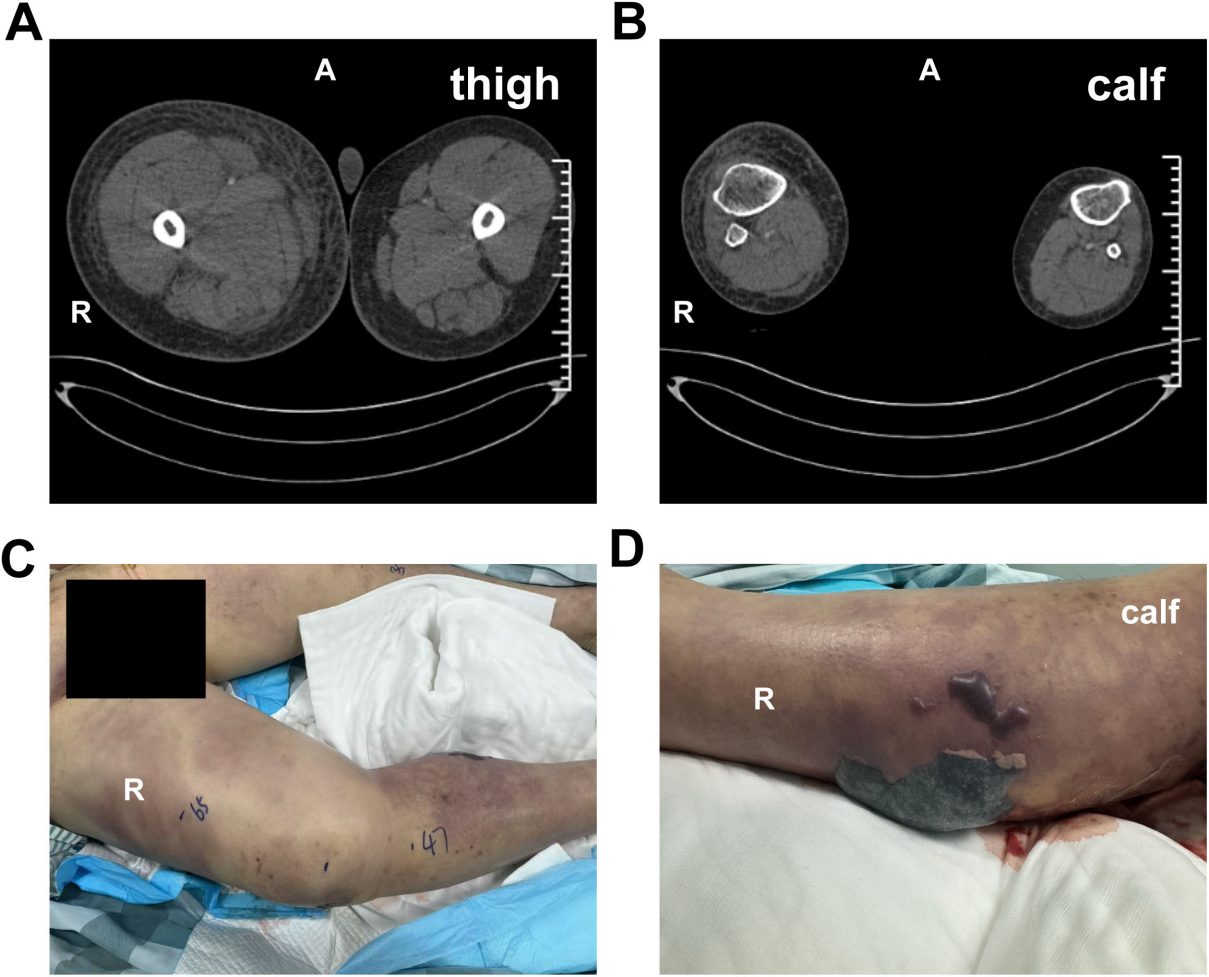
Preoperative imaging and clinical manifestation. Preoperative lower limb CTA revealed extensive subcutaneous fluid accumulation and fascial thickening in the right thigh (A) and right lower calf (B), with narrowed yet patent femoral, anterior tibial, and posterior tibial arterial flow tracts. Clinically, the right thigh (C) and right lower calf (D) showed diffuse erythema and subcutaneous ecchymosis; tense bullae were observed on the posterior aspect of the right lower leg (D).
